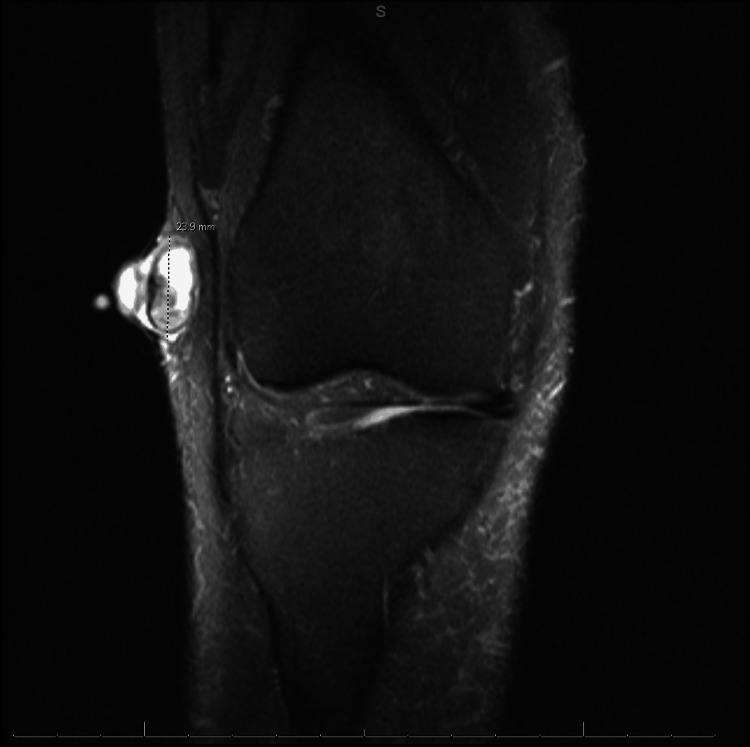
Coronal MRI (proton density-weighted, fat-suppressed, turbo spin echo) demonstrating the structure of the lesion along the lateral aspect of the right knee. MRI, magnetic resonance imaging
